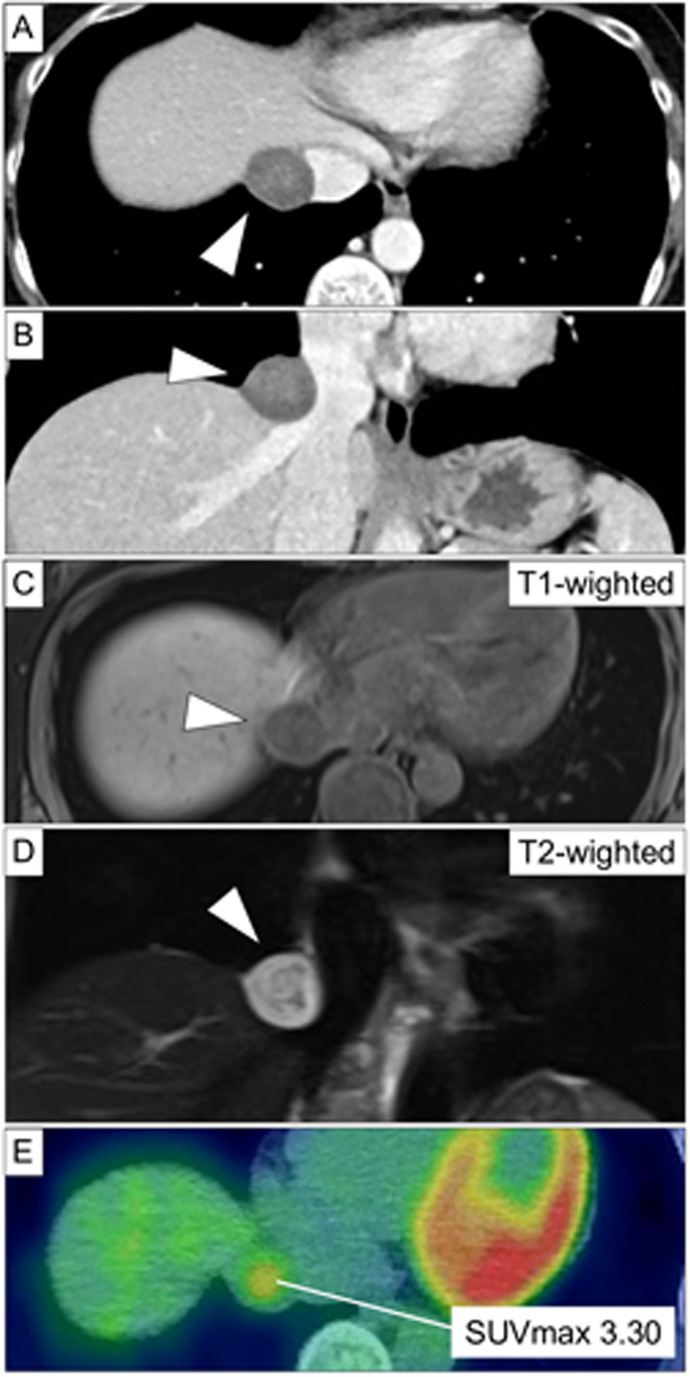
CT demonstrates a well-circumscribed but heterogeneously enhancing round tumor (arrowhead) compressing the suprahepatic inferior vena cava and right hepatic vein (A, B). MRI demonstrated a lesion (arrowhead) with high peripheral signal intensity on T2-weighted imaging, heterogeneous low internal intensity, and homogeneous signal intensity comparable to skeletal muscle on T1-weighted imaging (C, D). FDG-PET revealed mild uptake with a maximum standardized uptake value of 3.30 (E).
